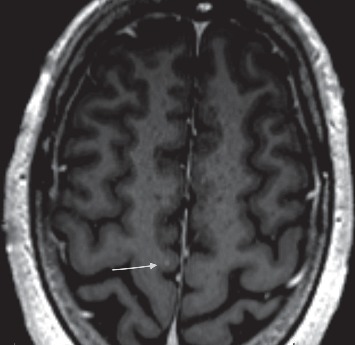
MRI Brain: T1 post-contrast sequence with an arrow pointing to an enhancing lesion over the right motor strip.
